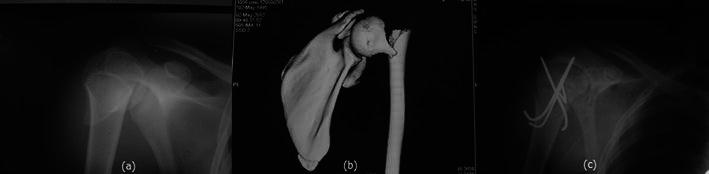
a Anteroposterior radiograph revealing the underlying surgical neck humerus fracture with minimal medio-lateral displacement. b CT scan image showing angulation with minimal comminution. c Postoperative radiograph showing achievement of good reduction with multiple Kirschner wires
